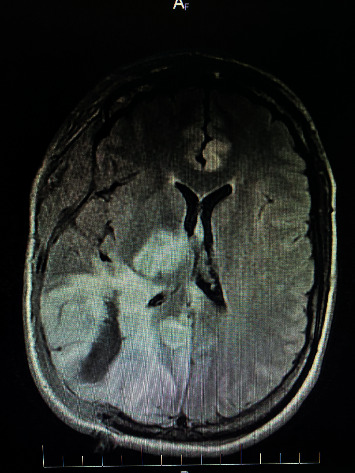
Magnetic resonance imaging (MRI) FLAIR axial head without contrast showing intraparenchymal hemorrhage status after craniectomy.
